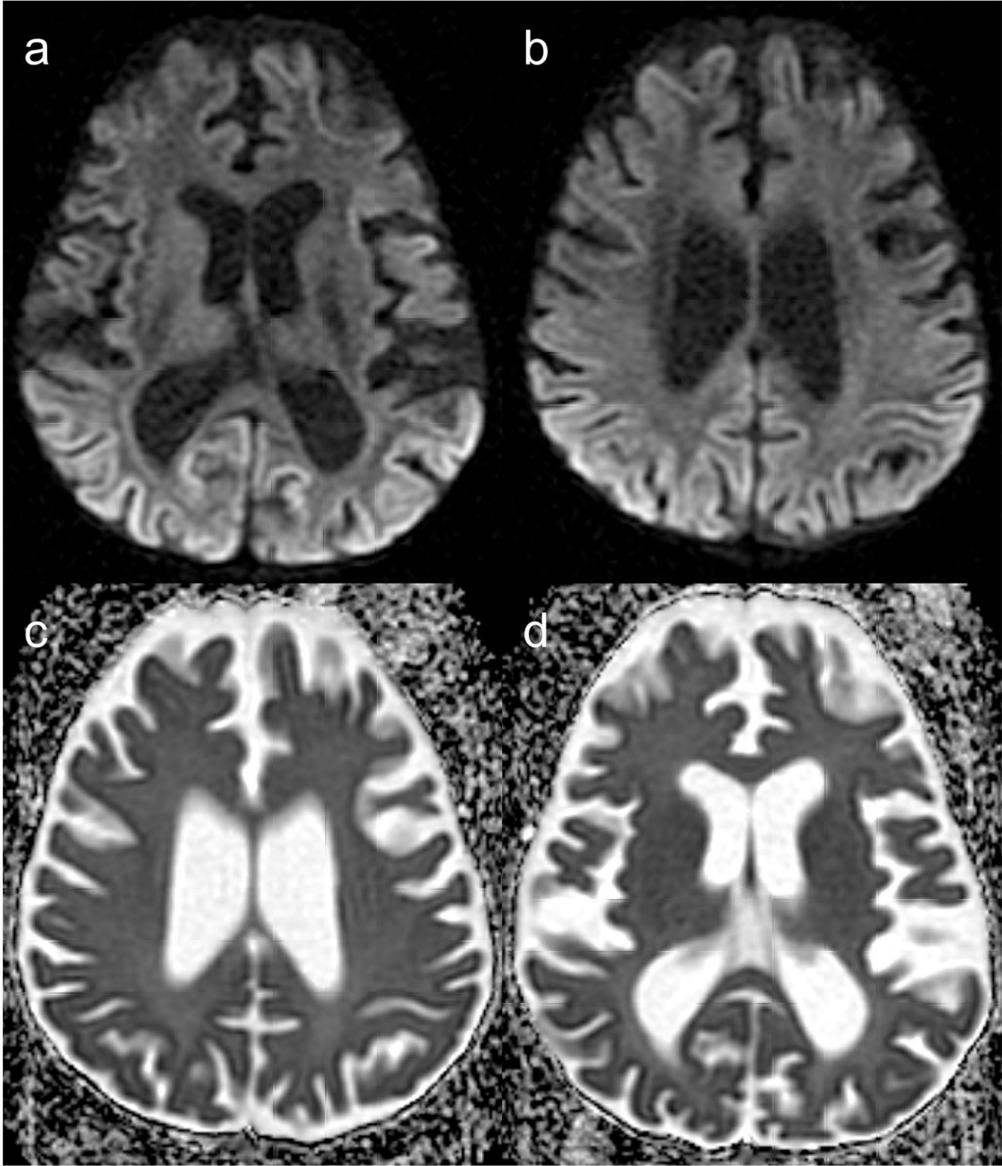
Severe combined immunodeficiency with no identified gene. A 2-year-old female patient with systemic JCV infection undergoing cidofovir treatment. Diffusion MR imaging (a and b – b1000, and c and d – ADC maps) depict extensive cortical areas of DWI hyperintensity and corresponding low signal on ADC maps, predominantly in the parieto-occipital regions. These findings have been sporadically reported in cases of encephalopathy secondary to lytic JCV infection of cortical pyramidal neurons. Diffuse cerebral atrophy is also present, likely related to prolonged immunosuppression for severe graft-versus-host disease following hematopoietic stem cell transplantation
